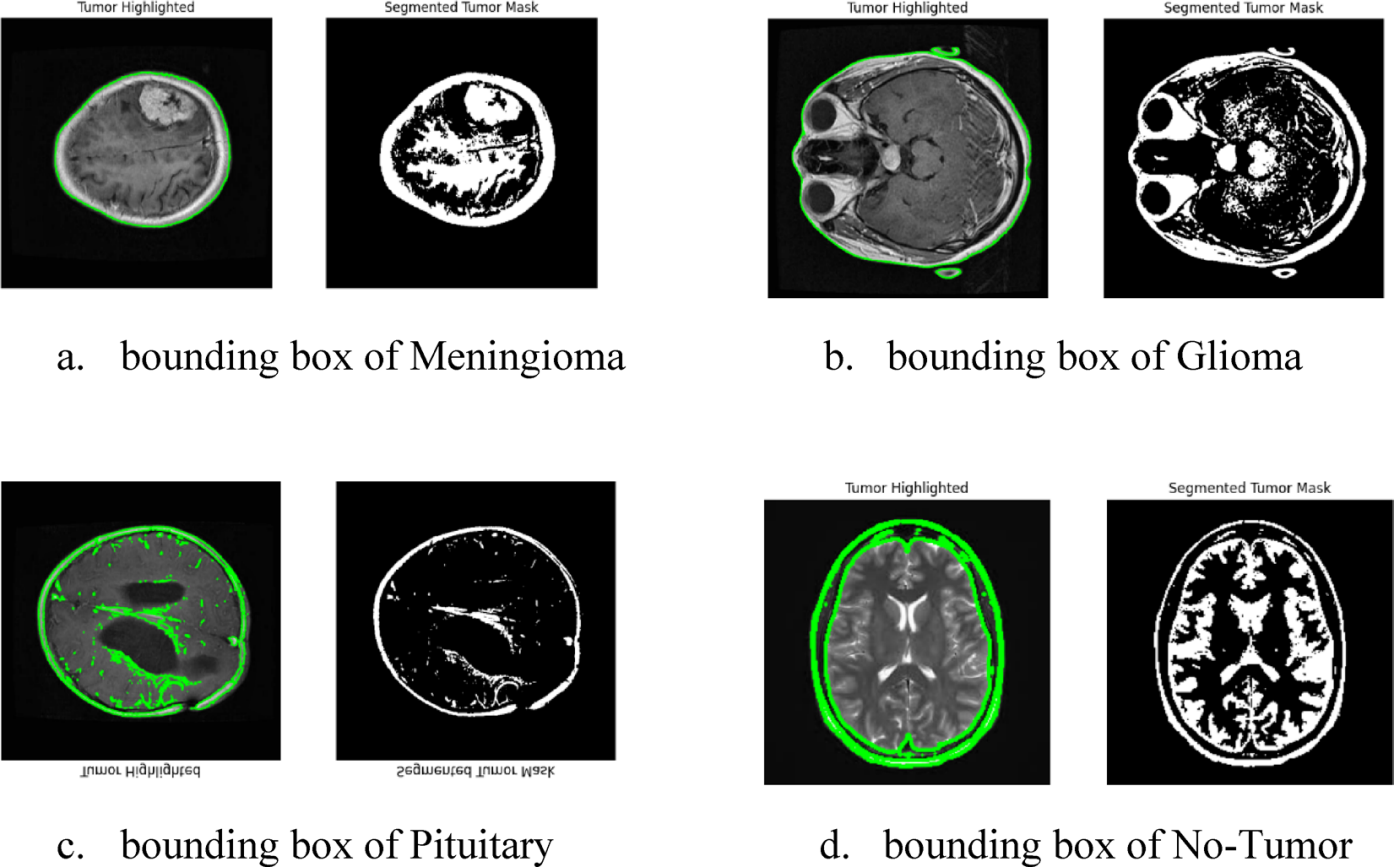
MRI images with bounding box and mask.
